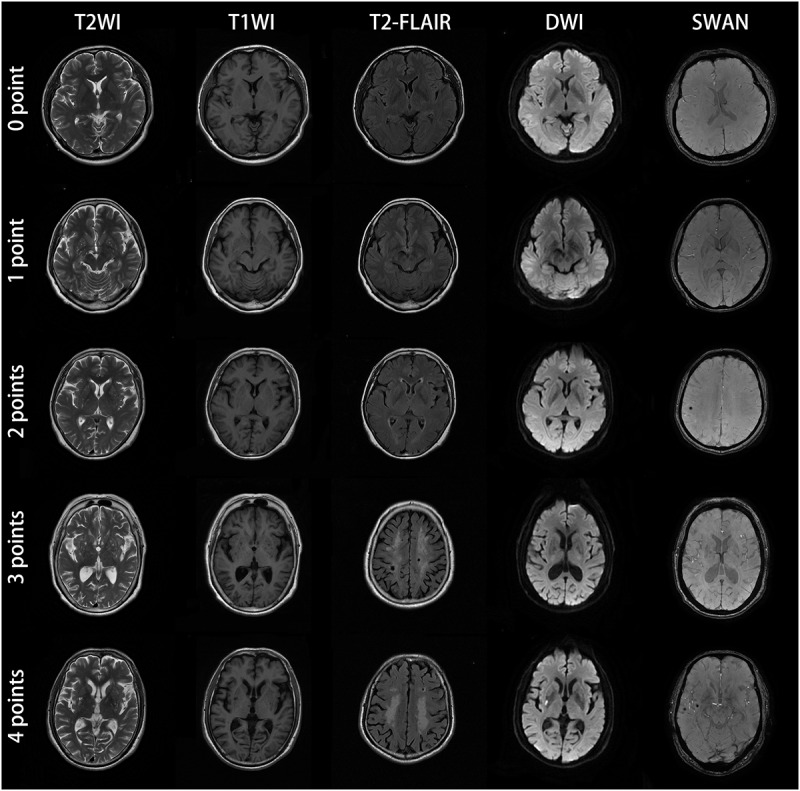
MRI images of subjects with different CSVD total burden score. The subject with score of zero point was a 57-year-old man with no abnormal lesions in MRI images. The patient with score of one point was a 61-year-old female, the bilateral basal ganglia represented punctate and linear lesions with diameter less than 3 mm, hypointensity on T1WI, hyperintensity on T2WI and low signal intensity on T2-FLAIR. The DWI and SWAN sequences showed no abnormality. The bilateral basal ganglia region coincided with EPVS (grade 2–3). For the patient with score of two points, bilateral basal ganglia showed dotted, linear cerebrospinal fluid signal shadow (diameter < 3mm), T2WI showed high signal intensity, T1WI showed low signal intensity, T2-FLAIR showed low signal intensity, which was consistent with the characteristics of EPVS. SWAN sequence of the right parietal lobe showed low signal intensity with diameter ≤ 10 mm, which conformed to the characteristics of CMBs. This CSVD patient was consistent with two points according to the criteria of total CSVD burden score. The patient with score of three points was a 60-year-old female. MRI showed EPVS (grade 2–3) in the bilateral basal ganglia, large confluent areas WMH (Fazekas 3 for DWMH) and lacunar infarction in the center of bilateral semioval center. SWAN sequences showed no abnormality. The patient with CSVD score of four points was a 62-year-old male. There were EPVS (grade 2–3) in the bilateral basal ganglia, large fused WMH (Fazekas 3 for DWMH) in the center of the bilateral semiovale center, acute lacunar infarction with high signal intensity in the right basal ganglia region in DWI image, and CMBs in the right temporal lobe. All the four kinds of imaging markers appeared in this patient.
